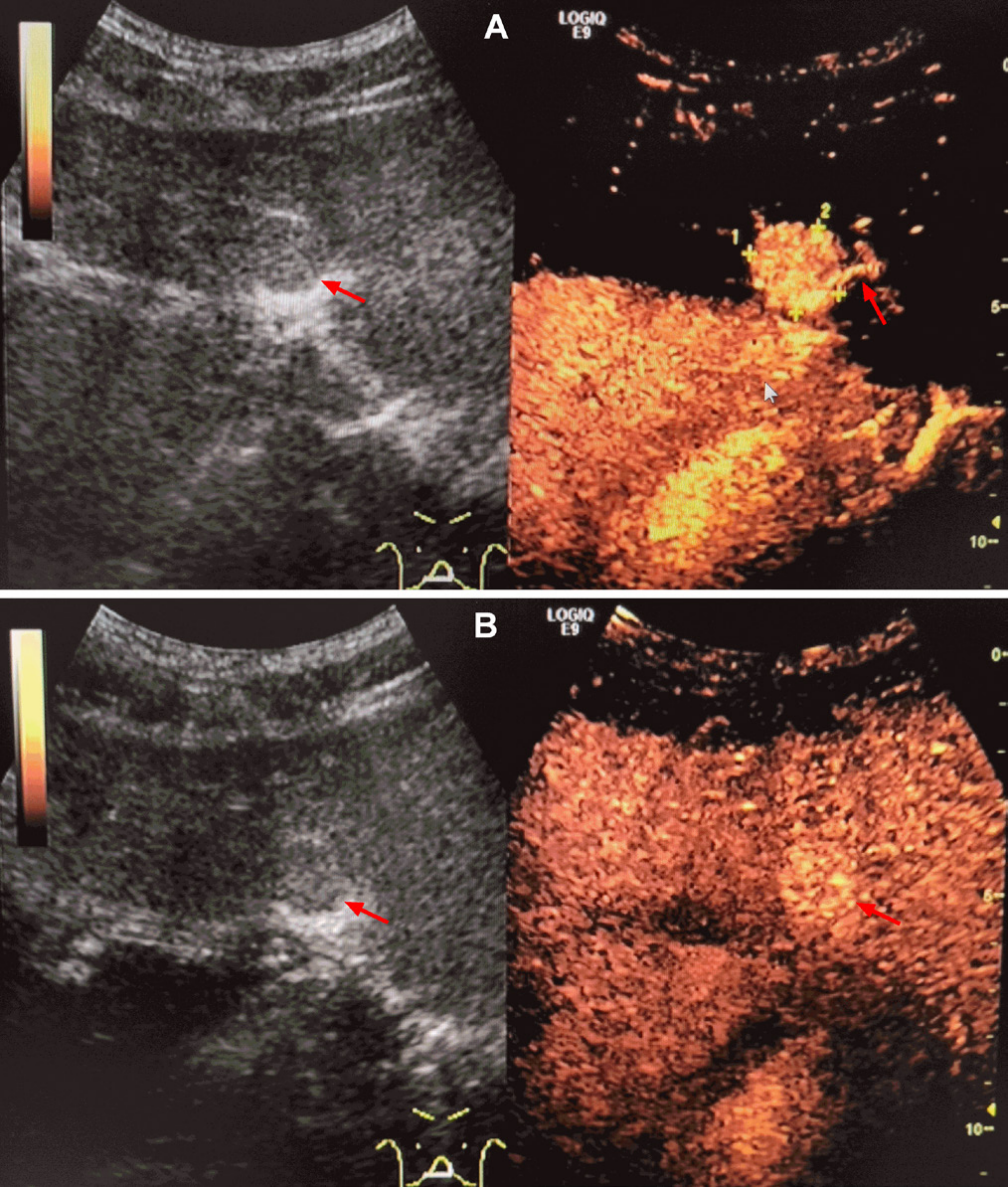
Ultrasound images of IHS in case two. The lesion in segment II was a homogenous isoechoic nodule (A, arrow) and had a size of 2.0 × 1.8 cm with an unclear boundary. Contrast-enhanced ultrasonography showed that the lesion exhibited a high enhancement in the arterial phase with a large vessel entering the lesion (A, arrow) and a continued slightly high enhancement in the sinusoidal phase (B, arrow).
